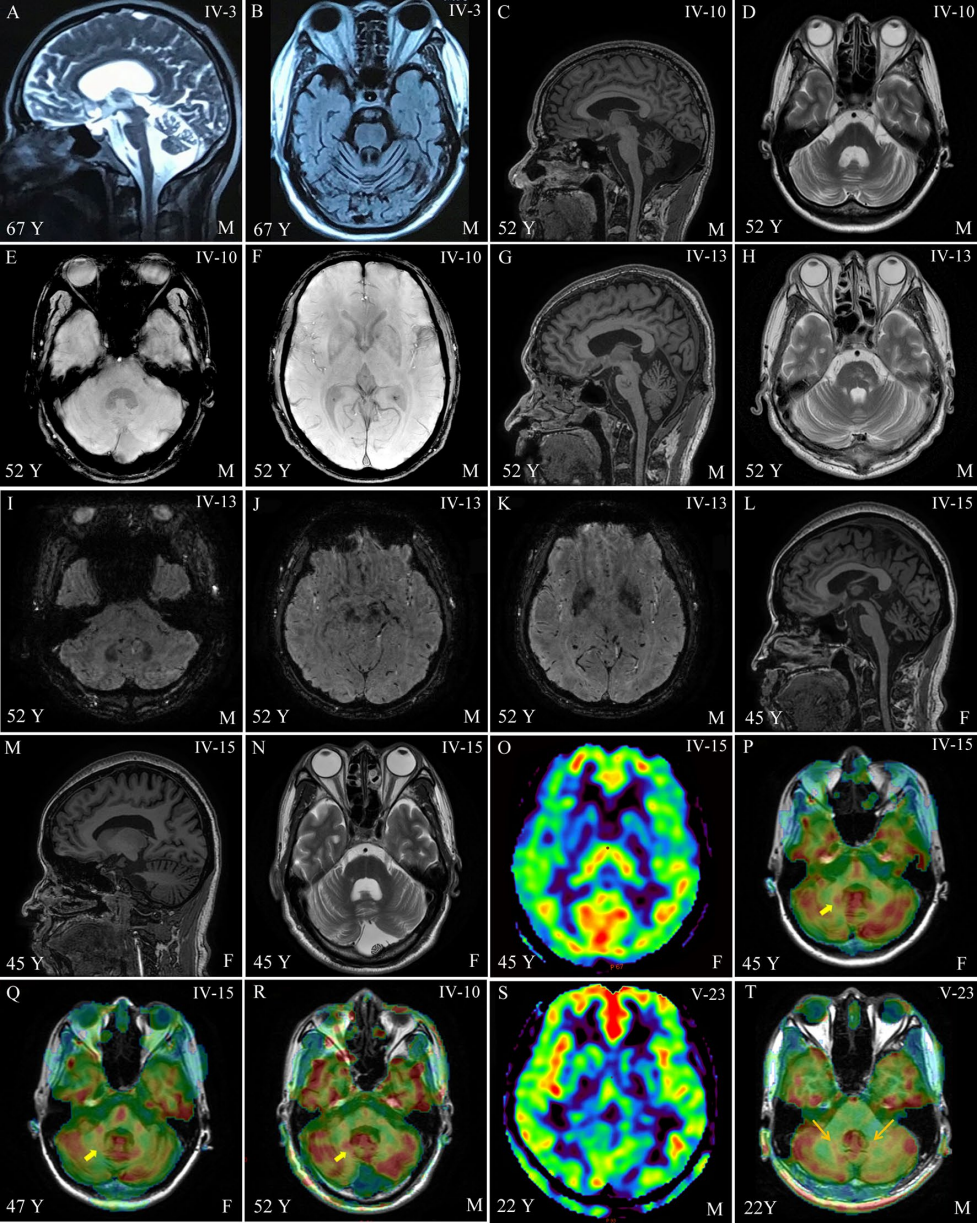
Magnetic resonance imaging in the brain. Brain MRI scans were performed on four clinically affected family members (IV-3, IV-10, IV-13, and, IV-15), and one asymptomatic member carrying the *CARS* mutation (IV-23). A Marked atrophy of the pons, vermis, and B cerebellar cortex is shown in MRI scans from member IV-3; C Atrophy of the pons, vermis, and D cerebellar cortex with a pontine-midline linear hyperintensity on T2WI is shown for member IV-10. E, F A normal iron-induced SWI hypo-signal in the dentate nuclei and basal ganglia is shown in MRI scans from member IV-10. G, H Mild atrophy of the pons, vermis, and cerebellar cortex with lacunas in the pons is shown in MRI scans from member IV-13. I–K An iron-induced SWI hypo-intensity in the dentate nuclei, red nuclei, SN, and basal ganglia is shown in MRI scans from member IV-13. L Marked atrophy of the vermis, pons, and L–N diffuse cerebellar atrophy with N a pontine-midline linear hyperintensity on T2WI is shown in MRI scans from member IV-15. O Decreased regional cerebral blood flow (rCBF) in the bilateral basal ganglia (from IV-15) and P in the cerebellar dentate nucleus, Q with further deterioration over two years, is shown in MRI scans from member IV-15. R Decreased rCBF in the cerebellar dentate nucleus (from member IV-10). S Decreased rCBF in the left putamen and the head of the caudate nucleus (from V-23), T but not in the cerebellar dentate nucleus.
